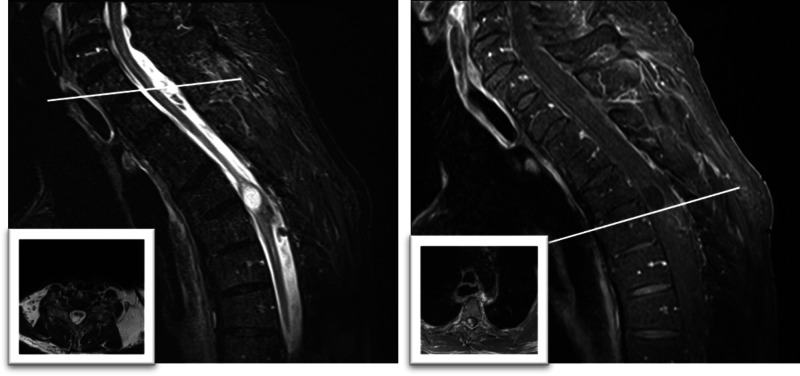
Preoperative Imaging Left: T2-weighted cervicothoracic MRI demonstrating an extra-axial lesion at the upper thoracic region and an intra-axial lesion in the midthoracic region. Inset: axial slice at the indicated level (C7-T1) demonstrating the extra-axial location. Right: T1-weighted cervicothoracic MRI with contrast of the same sagittal section as the image on the left, demonstrating heterogeneous contrast enhancement in the caudal lesion. Inset: Axial slice at the indicated level (T4-5) demonstrating the intra-axial location.
